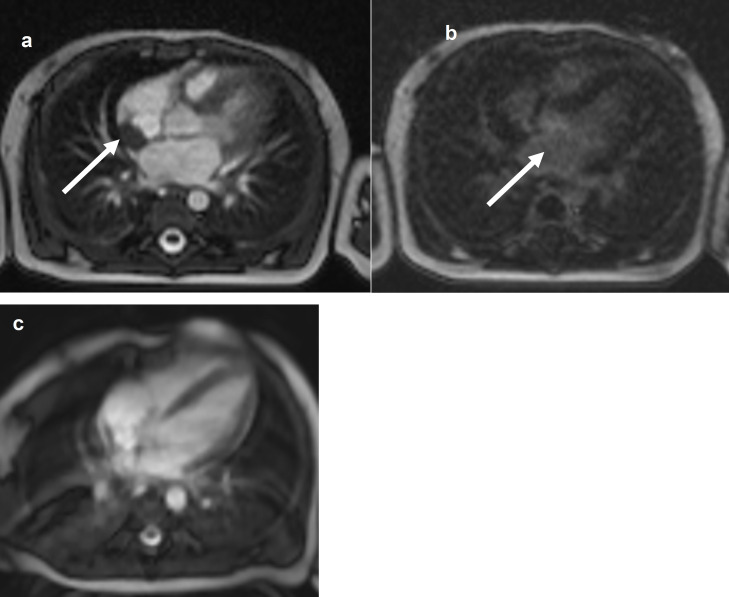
Cardiac-MRI comparison of intracardial mass (arrow) between the diagnosis and (a‒b) 20-months follow-up (c). T2 TrueFISP Sequence: Right intra-auricular mass at T2, attached to the posterior surface of the atrium and to the posterior part of the inter-atrial septum. Sequence after gadolinium injection: No lesion enhancement. Complete regression of intra-atrial mass.
